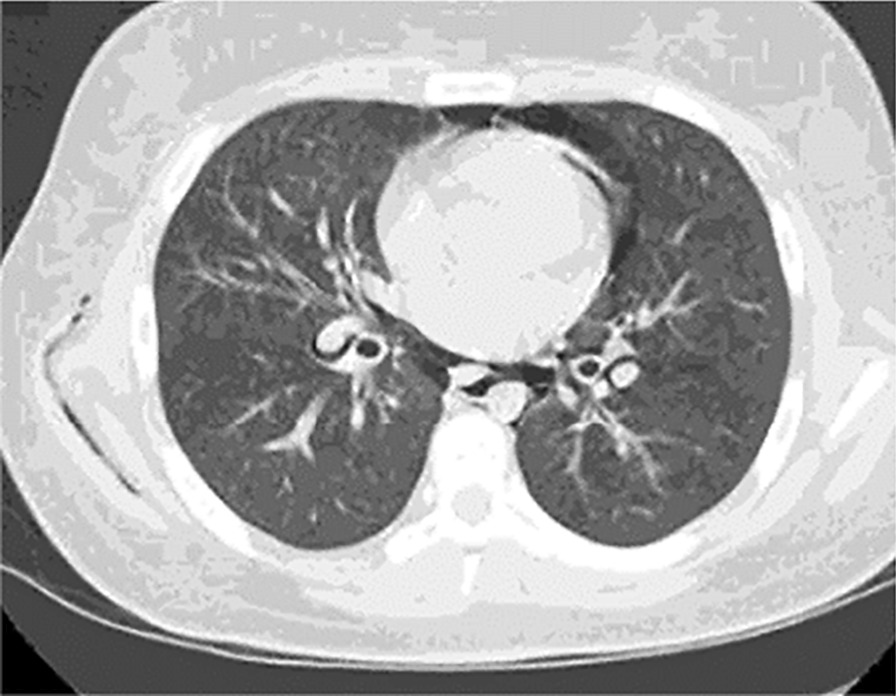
Chest computed tomography scan. Pneumomediastinum with modest aerial elevation which is distributed at the level of the peribronchovascular hilar and perilary spaces on both sides. No pneumothorax was identified
